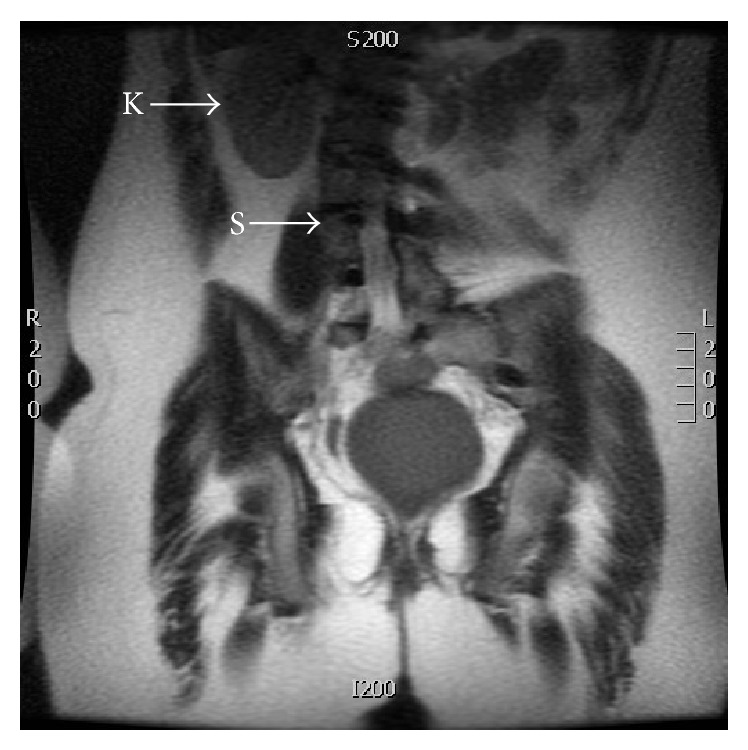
MRI of the lower abdomen and pelvis, demonstrating single kidney and scoliosis. Coronal T2 image, showing right Kidney (K) only with absence of left kidney and severe scoliosis (S).
